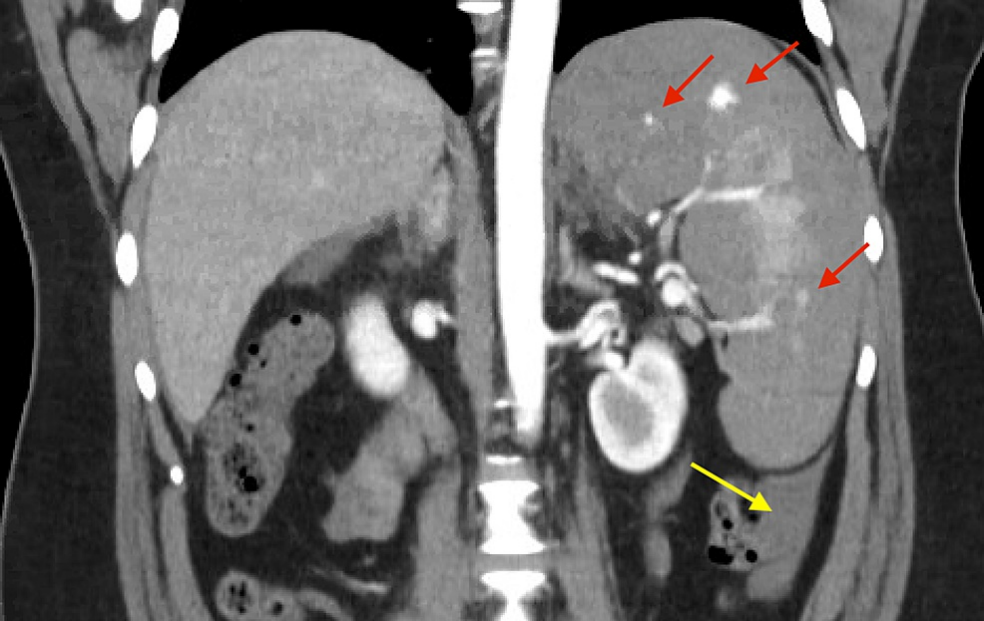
Coronal CT angiography of the abdomen demonstrated multiple areas of active extravasation within the spleen (red arrows). Perisplenic hematoma is observed with hemoperitoneum tracking along the left paracolic gutter (yellow arrow).
